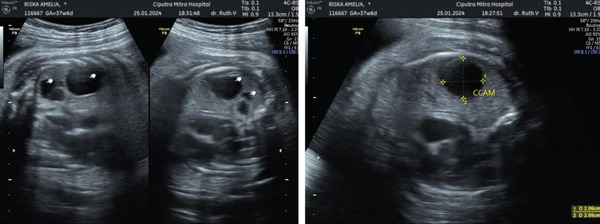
Initial ultrasound of suspected CCAM.
